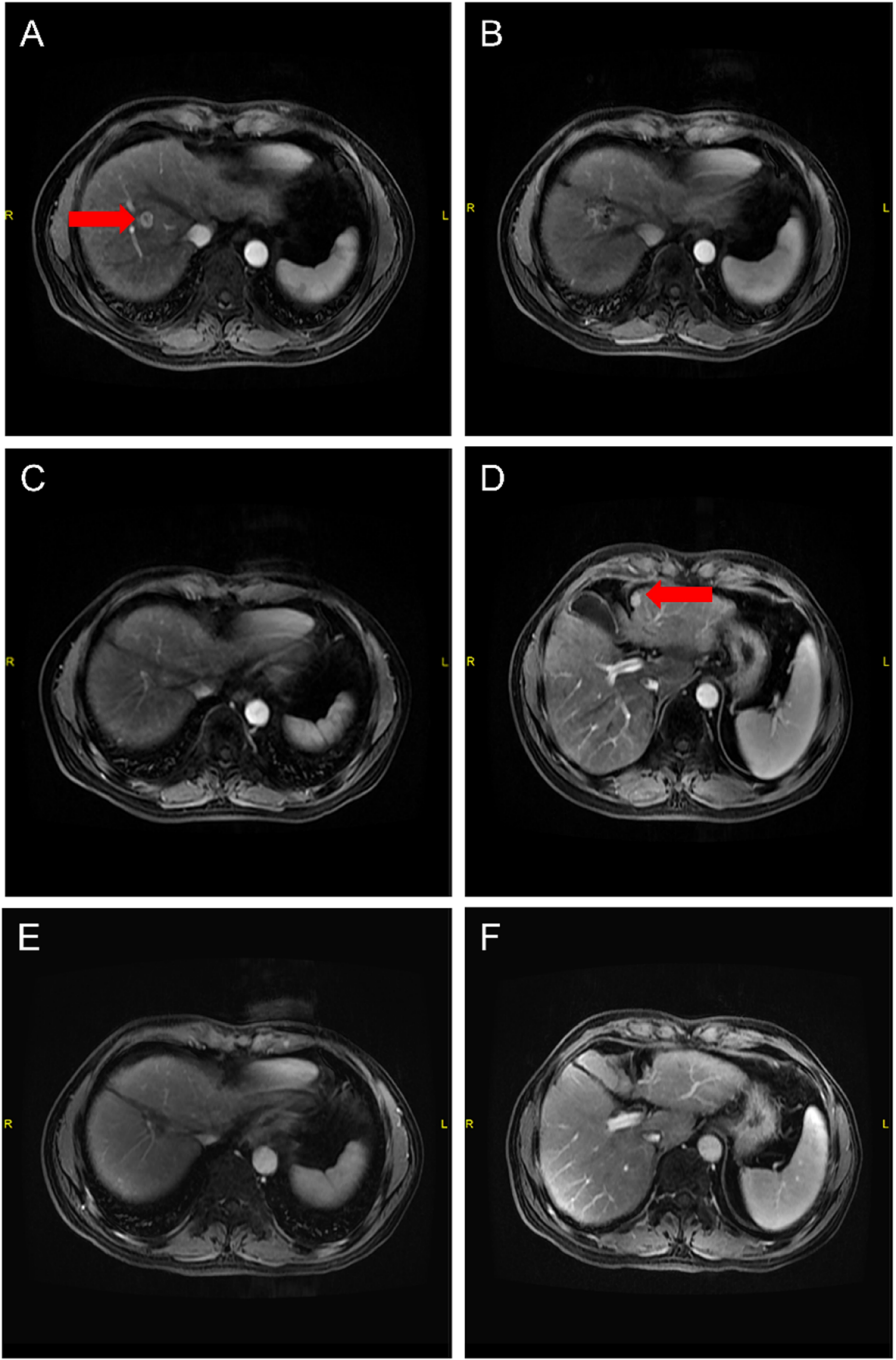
The patient who received two courses CK-SBRT, and the interval period was 26 months. October 2014: The primary abdominal MRI scan with the HCC lesion (in right liver) (a). Then the patient received CK-SBRT with 50Gy/5f. July 2015: MRI scan of lesion in right liver after CK-SBRT (b). December 2016: MRI scan of lesion in right liver showed the activity of lesion had disappeared (c). New lesion occurred in left liver (d), thereafter the patient received second-course CK-SBRT with 49Gy/7f. August 2019: MRI scan of 58 months after CK-SBRT (lesion in right liver) (e); MRI scan of 32 months after second-course CK-SBRT (lesion in left liver) (f)
